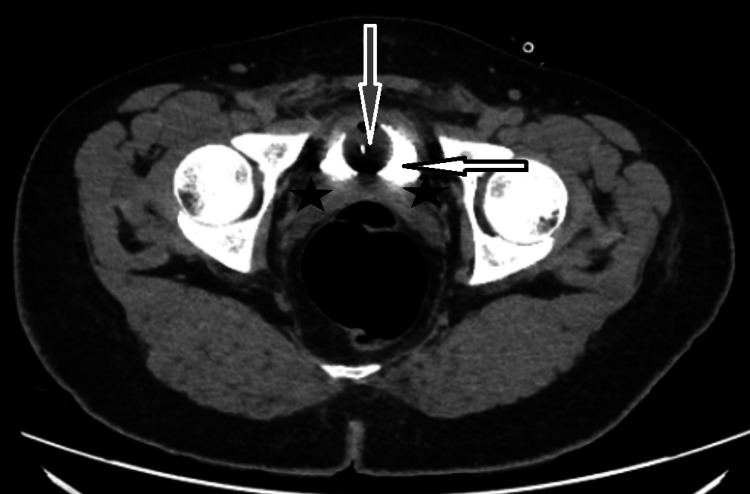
CT abdomen axial view (20-minute delay sequence) day 3 after surgery showing the bladder with contrast (white arrow) and a Foley catheter (grey arrow) in place with no contrast leak in the peri-vesical area (black stars). White arrow: urinary bladder with contrast, gray arrow: Foley catheter, black stars: peri-vesical area with no contrast leak CT: computerized tomography
